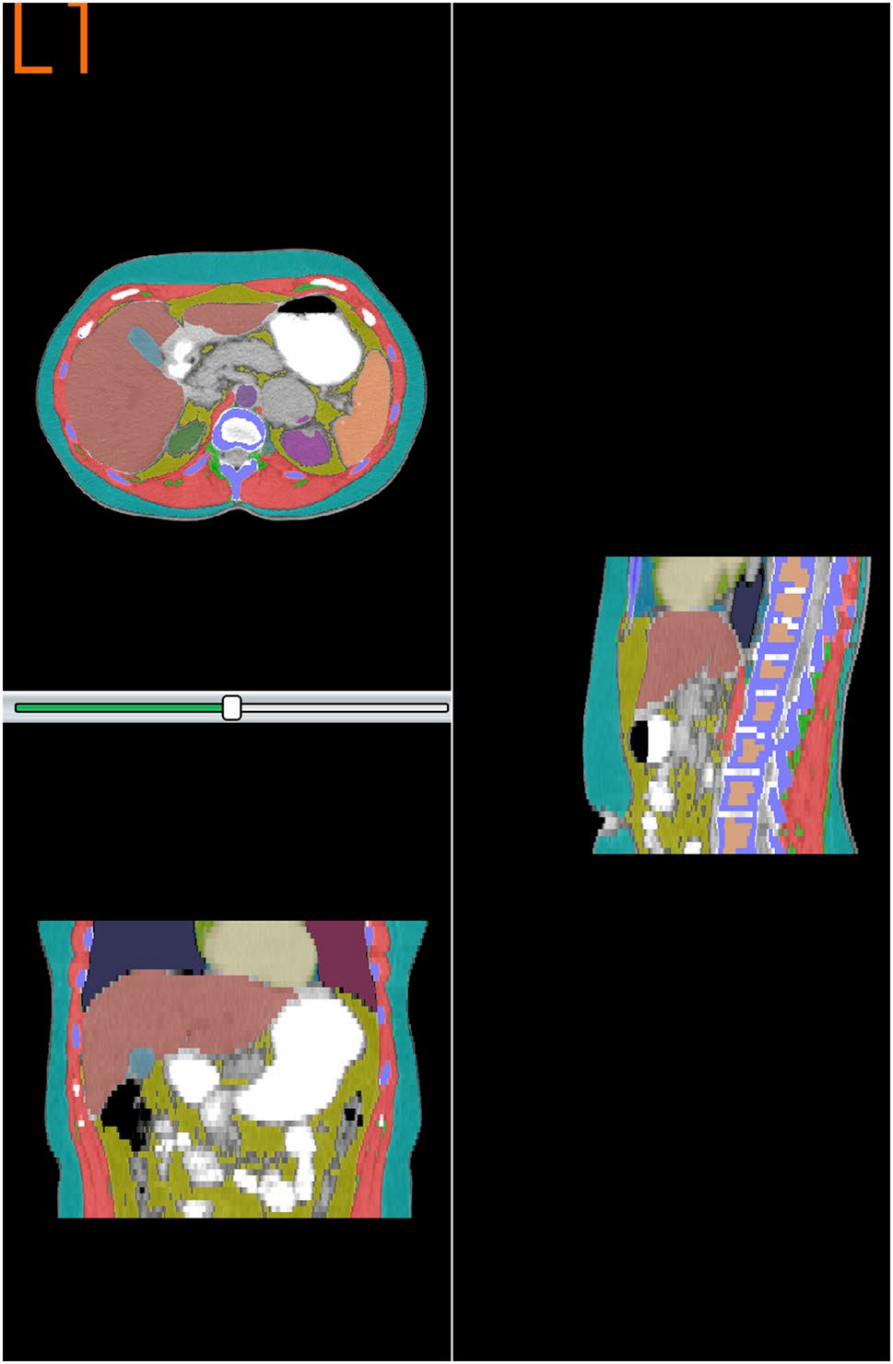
Axial CT scan image at L1 with automated body composition in a 35-year-old woman with ACC.
